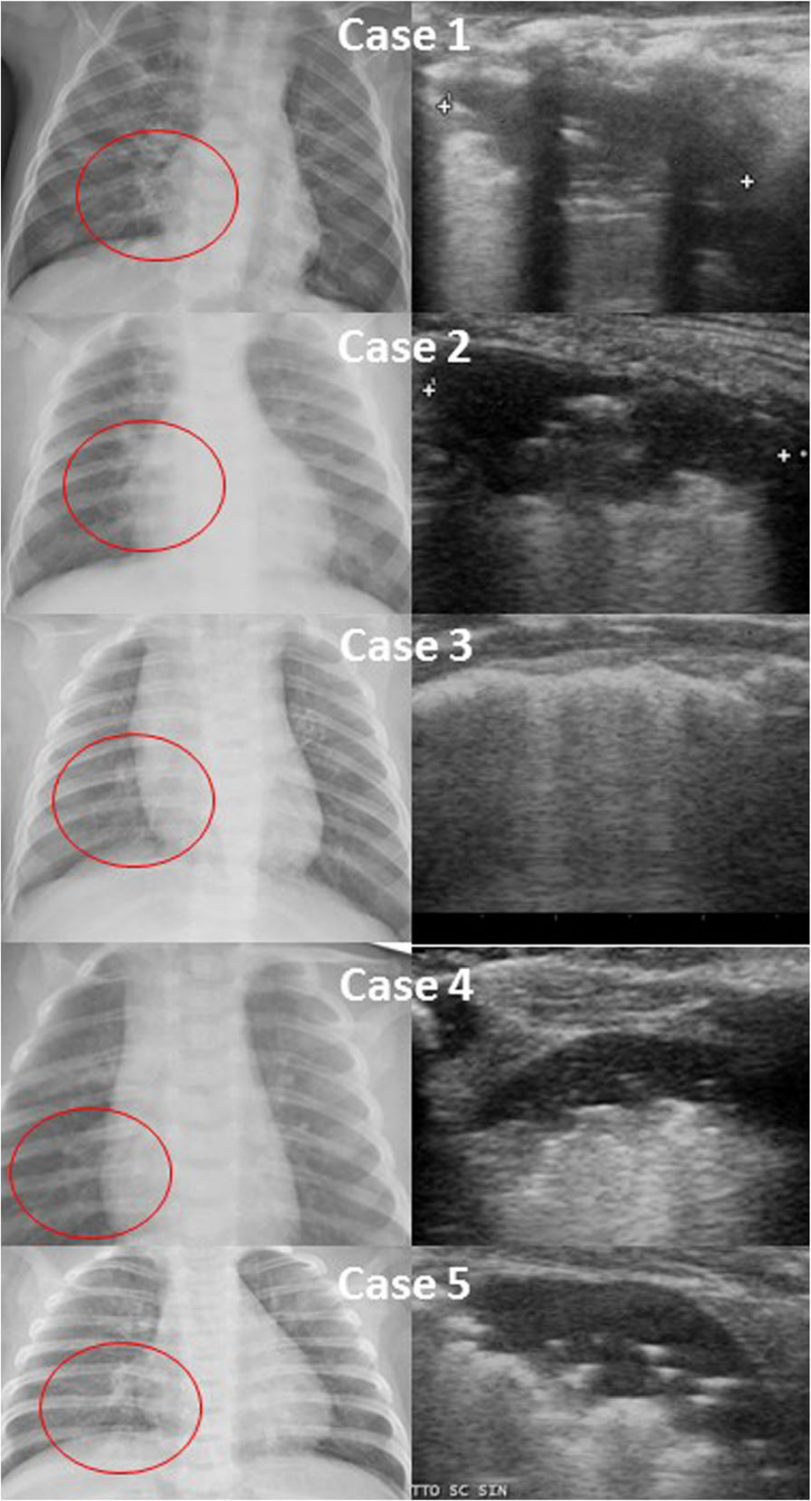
A case series of paracardiac consolidations on chest X-ray and the corresponding pattern on lung ultrasound, showing posterior, paravertebral, subpleural consolidations
